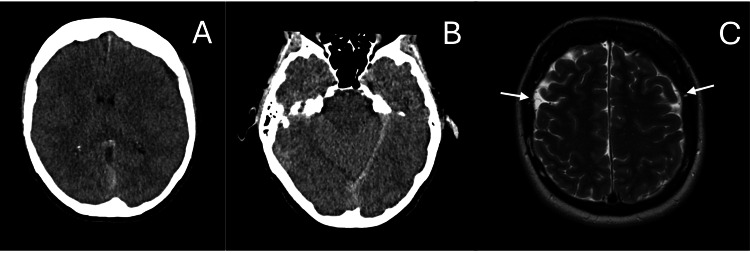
Axial non-contrast CT brain demonstrating a shallow left parafalcine subdural hematoma (A) with extension to the left tentorium cerebelli (B). T2-weighted axial MRI sequence showing bilateral shallow subdural collections (C) (white arrows).
